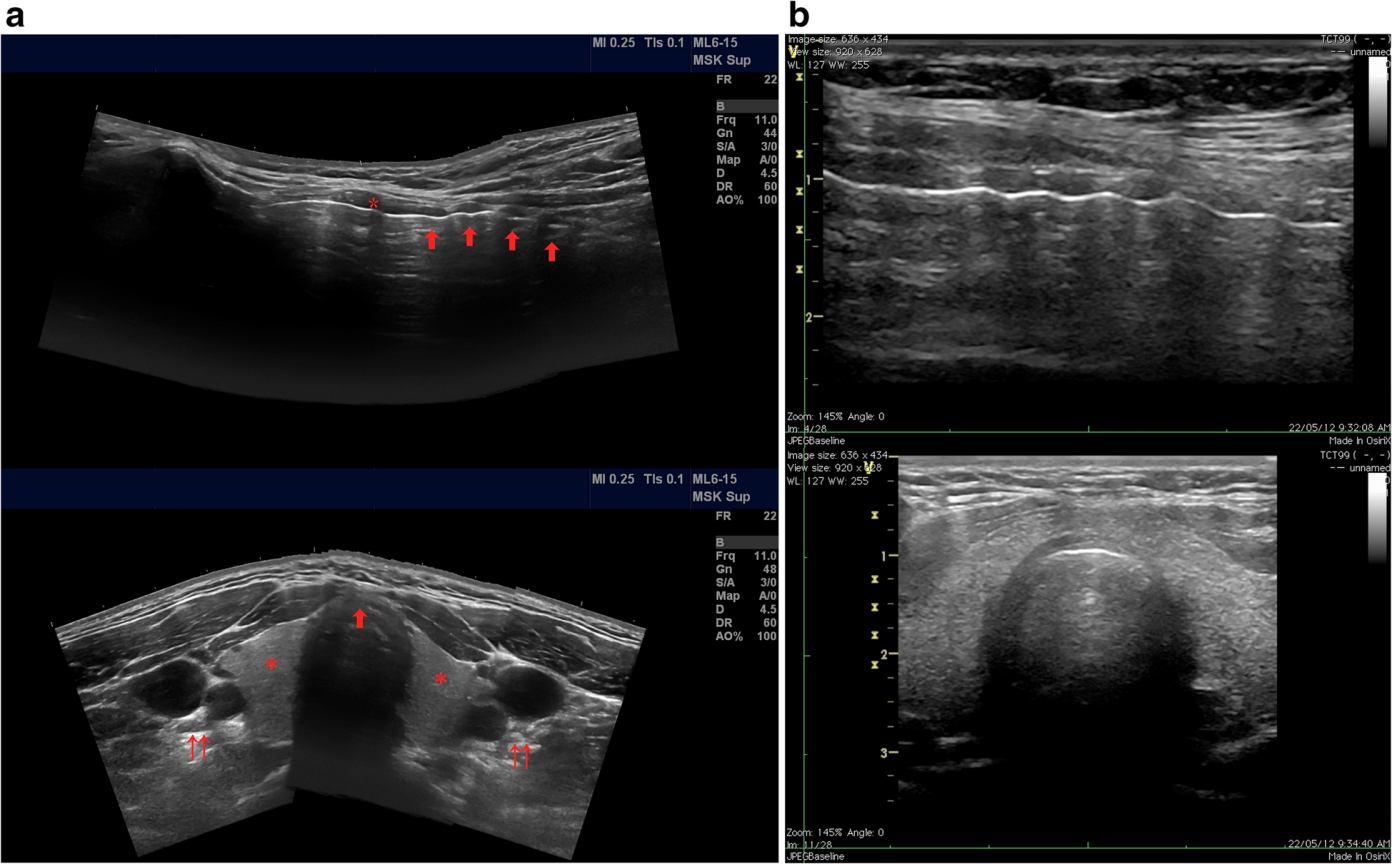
Ultrasound anatomy of the neck. (a)
